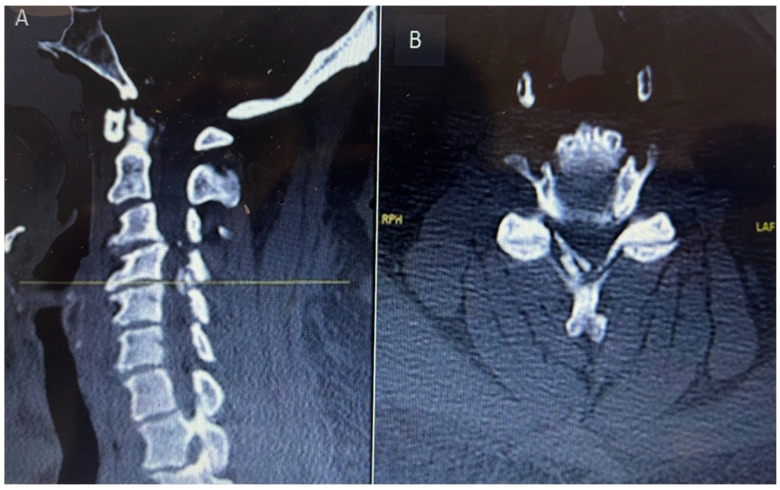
Preoperative sagittal (A) and axial (B) CT cervical spine showing calcific deposit at the cervical 4 level causing spinal stenosis.
